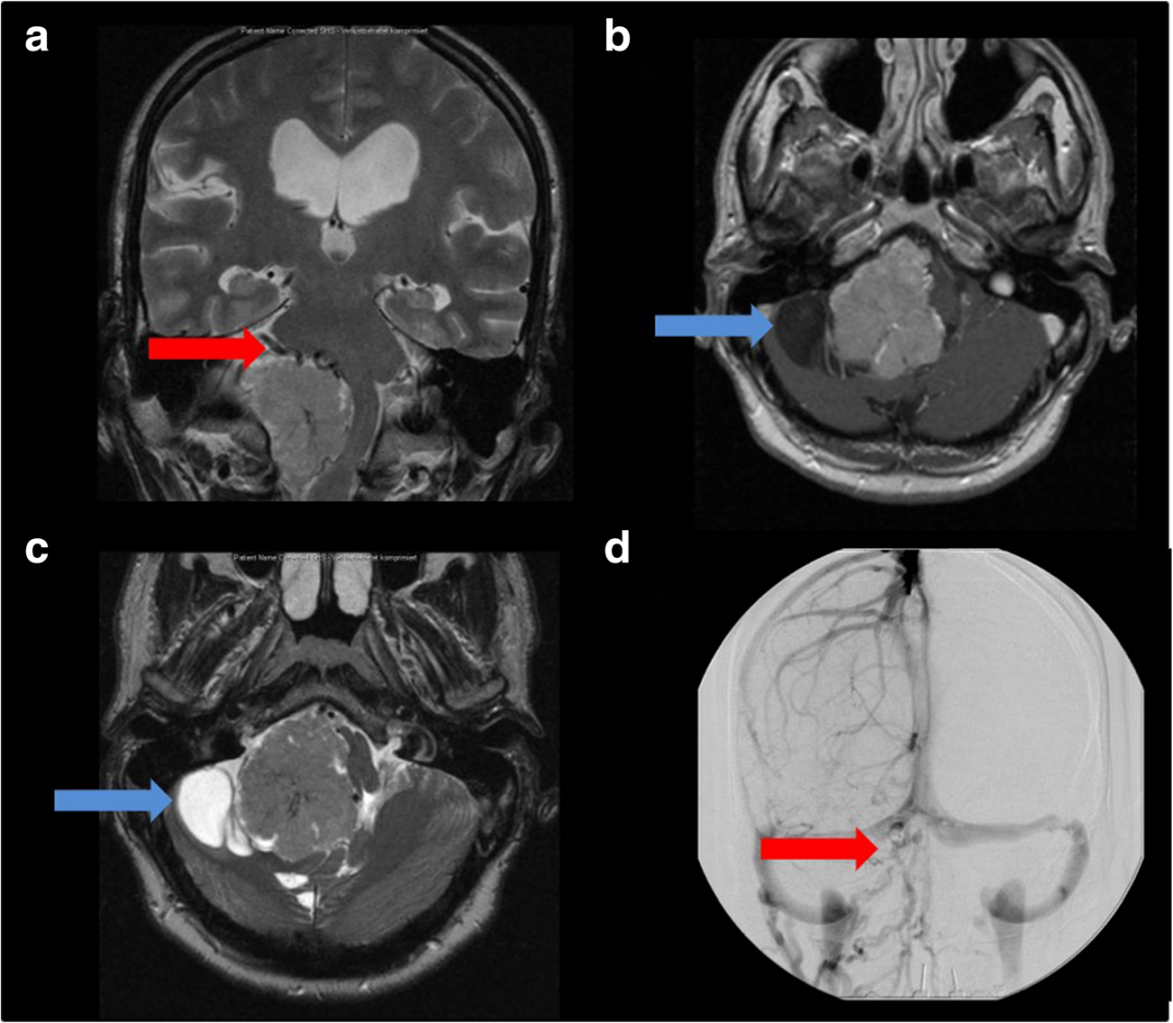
Coronal (A) and axial (B + C) MRI of type 3 choroid plexus papilloma: tumor portions are present in the cerebellopontine angle (CPA) and cerebellomedullary angle (CMA) (tumor portions superior and inferior to the line between the pons and medullar oblongata); angiography (D) revealed that the blood supply to the tumor arose from the right anterior inferior cerebellar artery and right posterior inferior cerebellar artery
